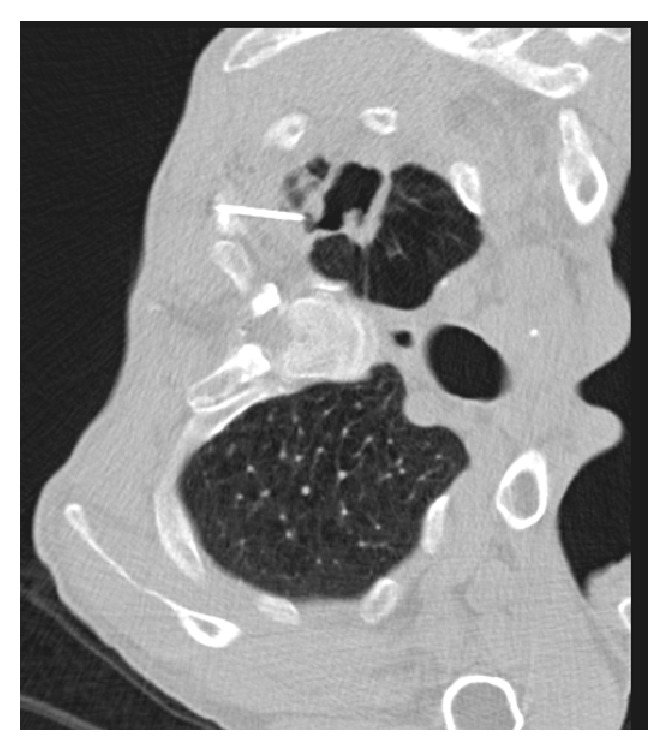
CT image obtained during lung biopsy with the patient in left lateral position and the tip of the guiding needle in the wall of a large cavitary lesion of the right upper lobe.
